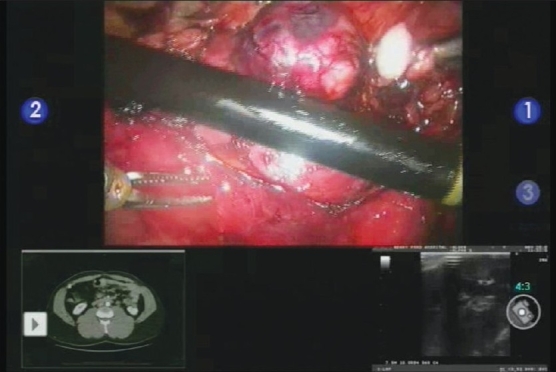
Use of TilePro during a robotic partial nephrectomy. TilePro image demonstrating the ability to simultaneously view live intraoperative ultrasound image (right lower corner) and preoperative CT image (left lower corner) on the console screen during a robotic partial nephrectomy. TilePro is being used to delineate tumor margins prior to tumor excision
